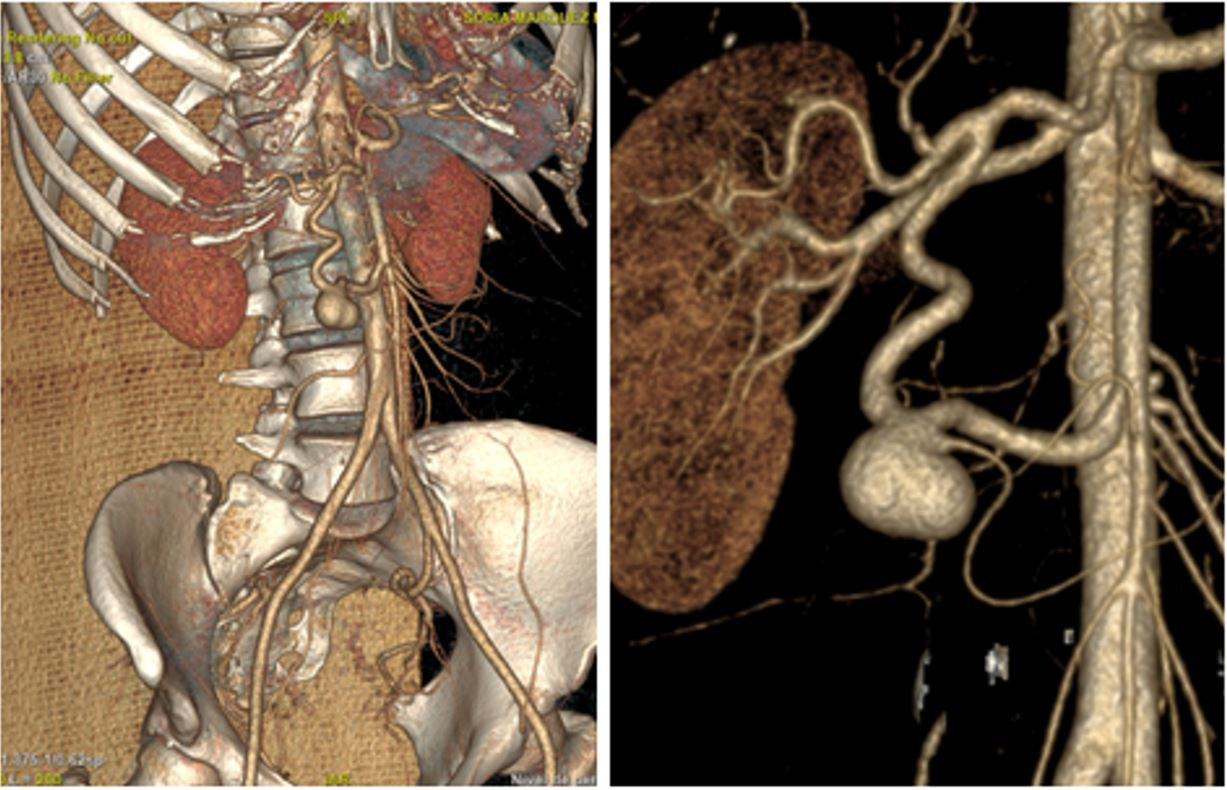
Computed tomography (CT) scan of the abdomen.
